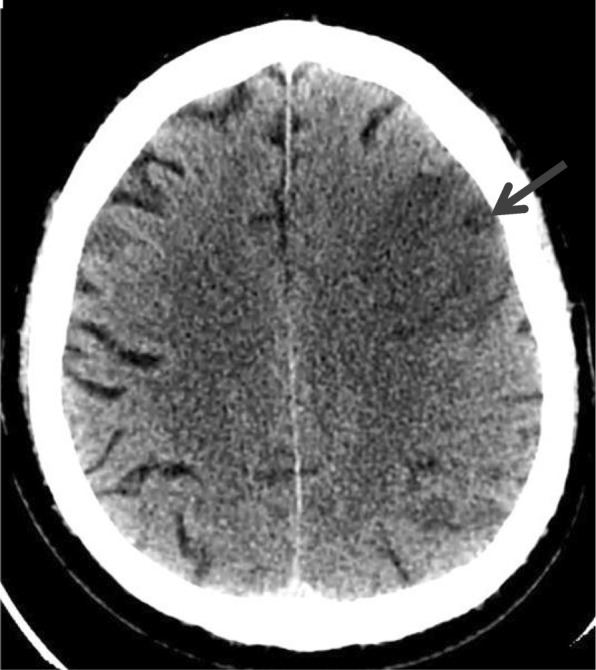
Case3: Non-enhanced cranial CT showed subacute ischemic lesion in the area of the left middle cerebral artery
